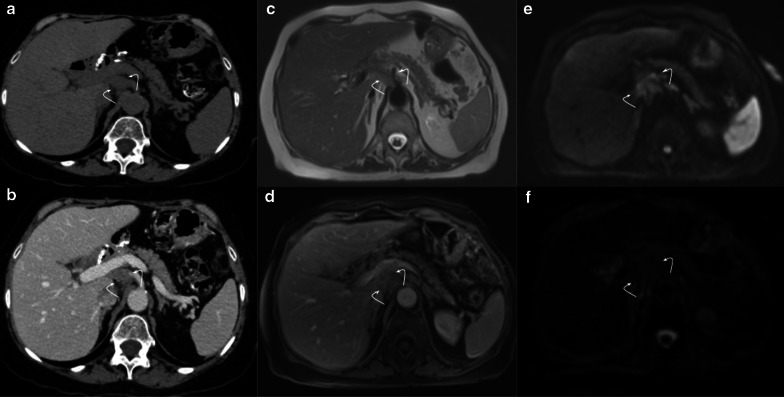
Tumor recurrence. DWI value. 69-year-old female patient during routine follow-up after pancreaticoduodenectomy due to pancreatic adenocarcinoma. (a, b) Non-contrast and portal venous phase CT scan, respectively. Shows an ill-defined soft tissue enhancing image in the surgical bed (curved arrows). (c, d, e, f) Six month later MRI control. Persistent image surrounding the celiac trunk with no significant progression (curved arrows in c and d). DWI (e) and ADC map (f) shows restriction, raising local recurrence diagnosis (curved arrows in e and f). The patient evolved unfavorably with disease progression
